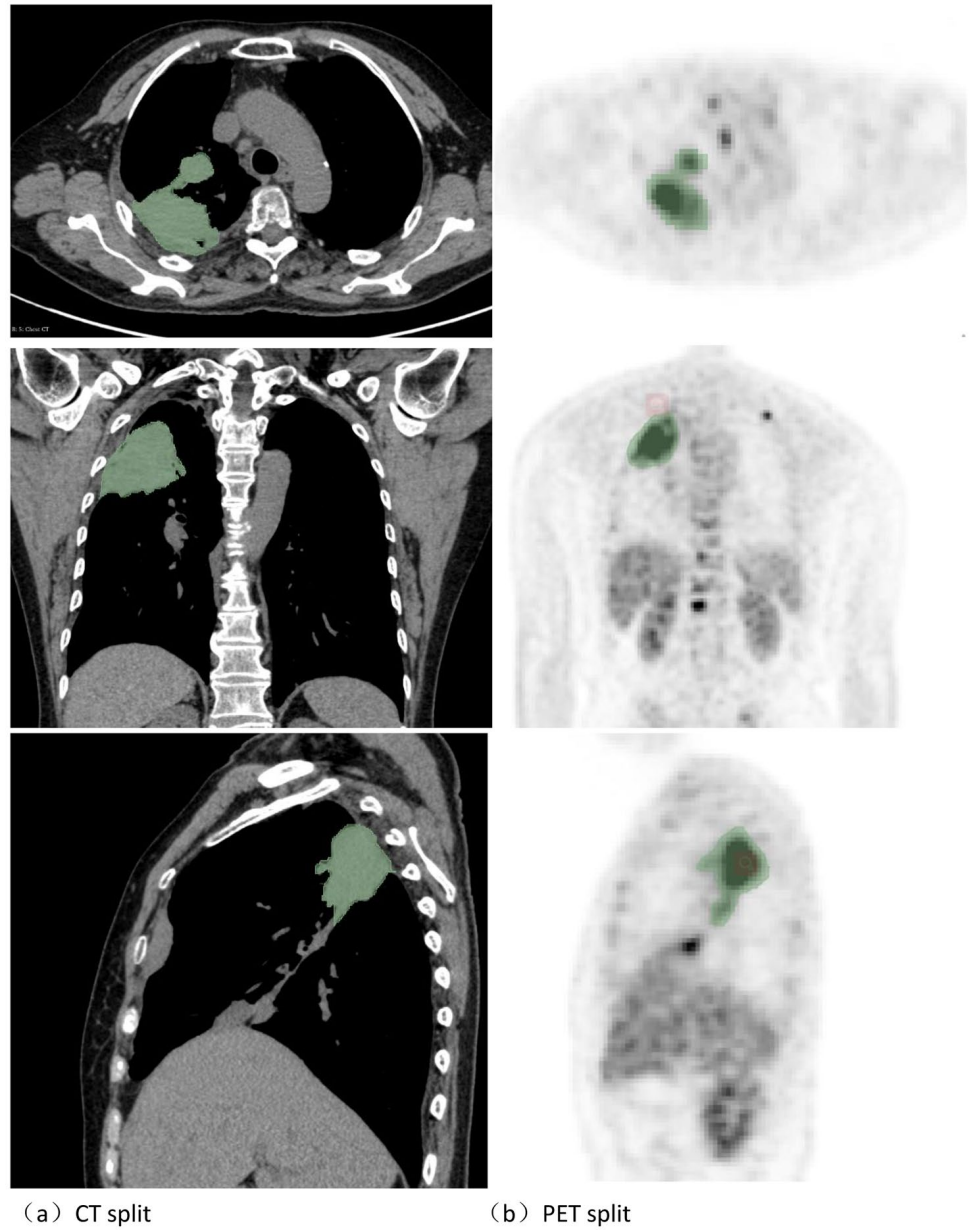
Irregular abnormal density foci in the upper lobe of the right lung. Fig(a): Plain CT scan of adenocarcinoma of the upper lobe of the right lung to sketch the image;Fig(b) PET sketching image of adenocarcinoma in the upper lobe of the right lung
